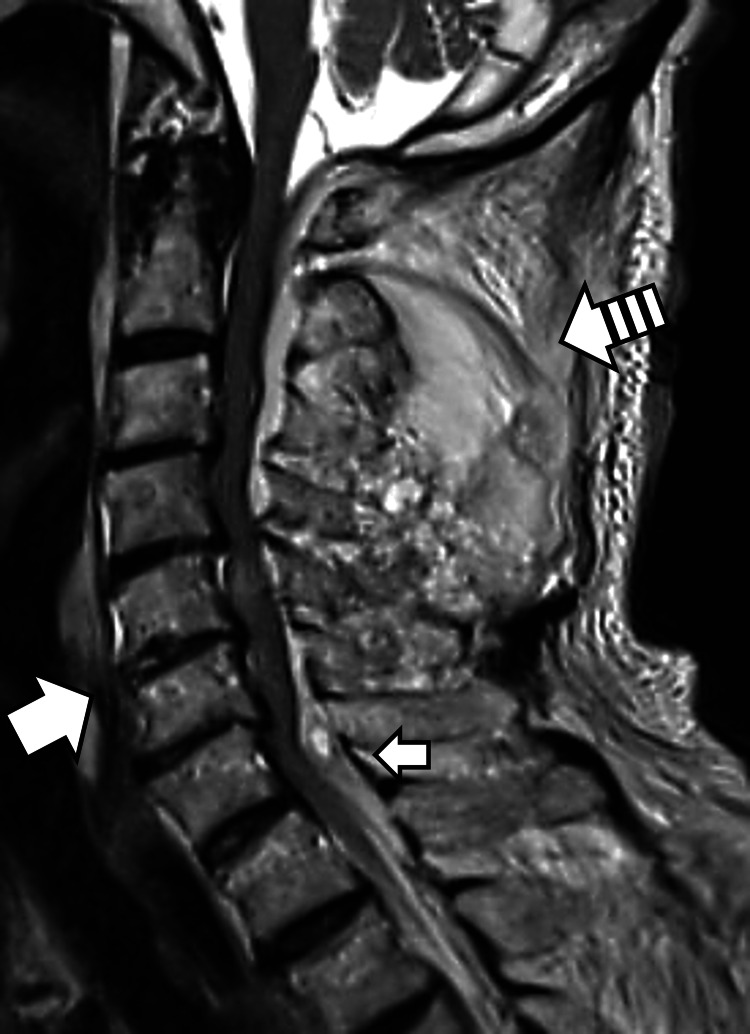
Sagittal T2-weighted magnetic resonance image (MRI). The thick arrow indicates anterior cervical hematoma. The thin arrow highlights the epidural hematoma. The striped arrow shows a soft tissue hematoma in the upper cervical spine.
